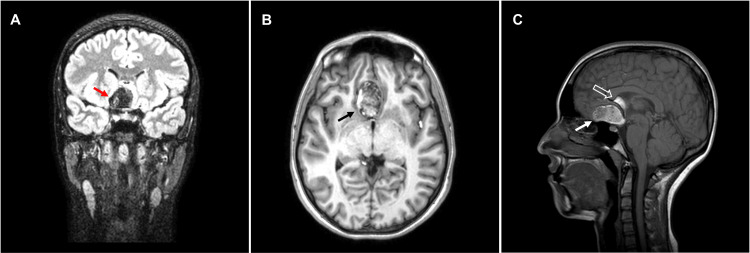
Preoperative MRI (A) Coronal FLAIR at the level of the pituitary stalk showing the suprasellar dermoid cyst (full arrow), (B) axial T1 view of the cyst (full arrow), and (C) sagittal T1 view showing both the suprasellar dermoid cyst (full arrow) and the subrostral corpus callosum fatty component (empty arrow) FLAIR: fluid-attenuated inversion recovery
